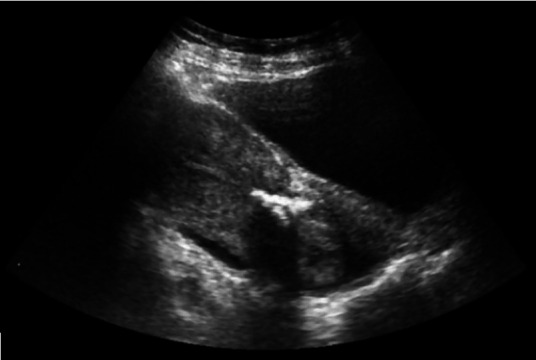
Pelvic Ultrasound showing heterogenous & hyperechoic content with multiple calcific foci within the endometrial cavity.
